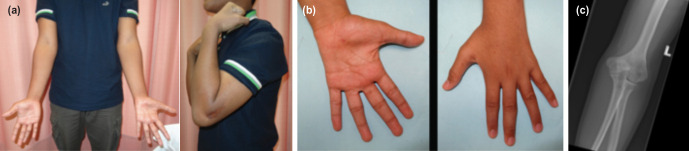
Clinical and radiological photographs four years after surgery. (a) Left elbow deformity corrected with full range of elbow movement. (b) Resolution of the claw hand deformity. (c) Radiograph showing complete healing with a fish-tail deformity of the distal humerus.
